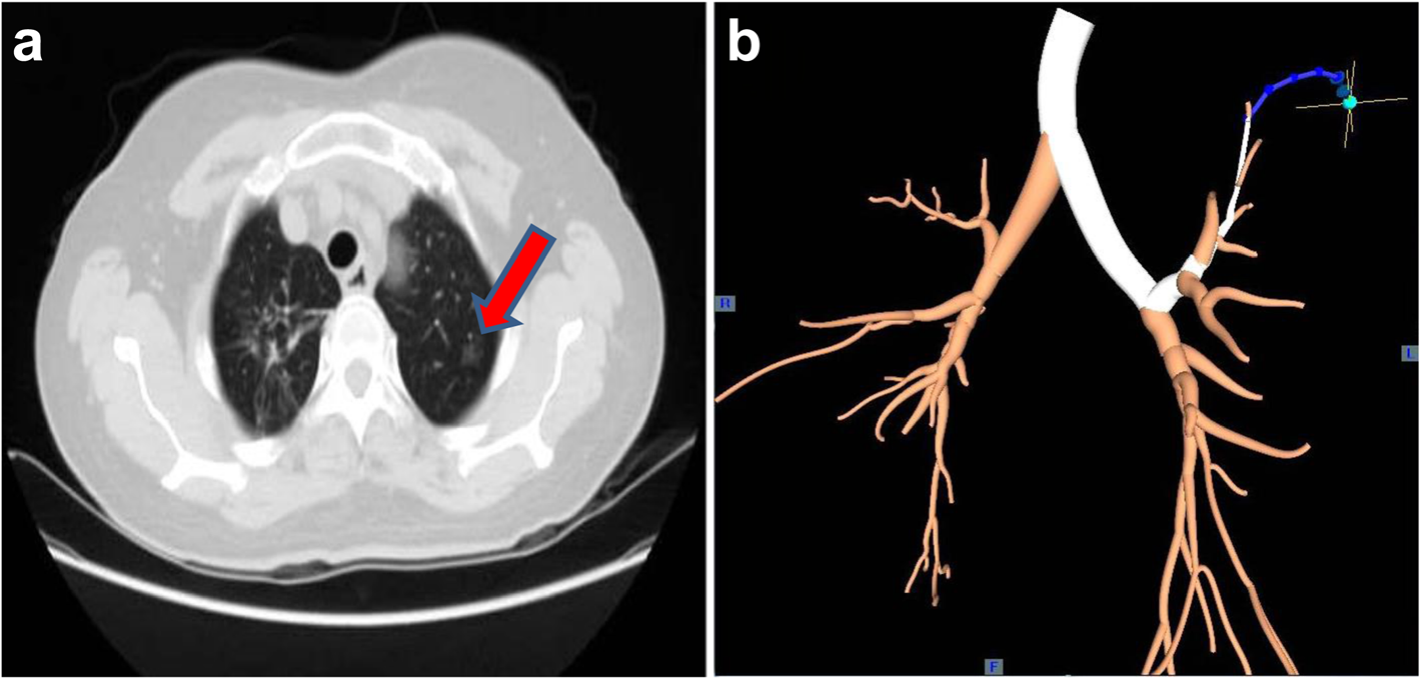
Preoperative preparation. a Preoperative CT scan was carried out to determine the location of the lesion; the red arrow shows the lesion. b Preoperatively establish the navigation path by ENB, in which the green point is a lesion
